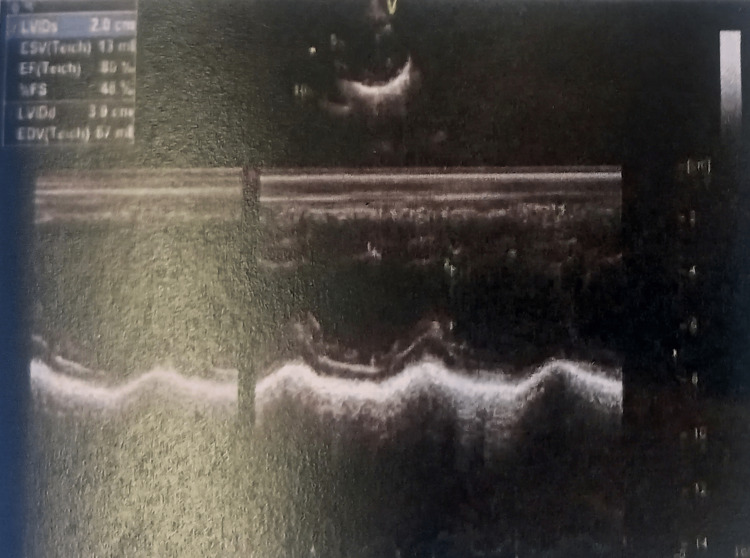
M-mode echocardiogram of the patient with mitral valve stenosis
